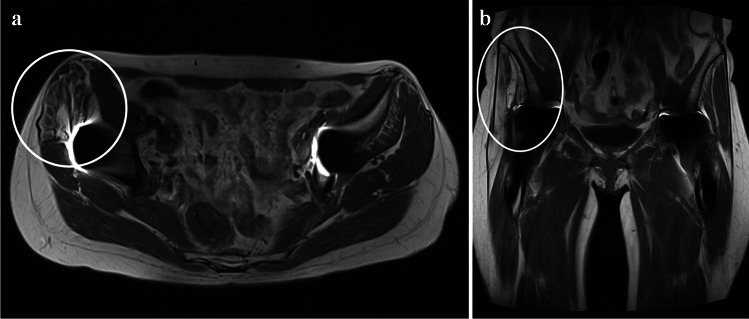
MRI showing right-side gluteus medius atrophy and Goutallier 4 fatty degeneration; T1-weighted sequences in a the axial and in b the coronal plane
